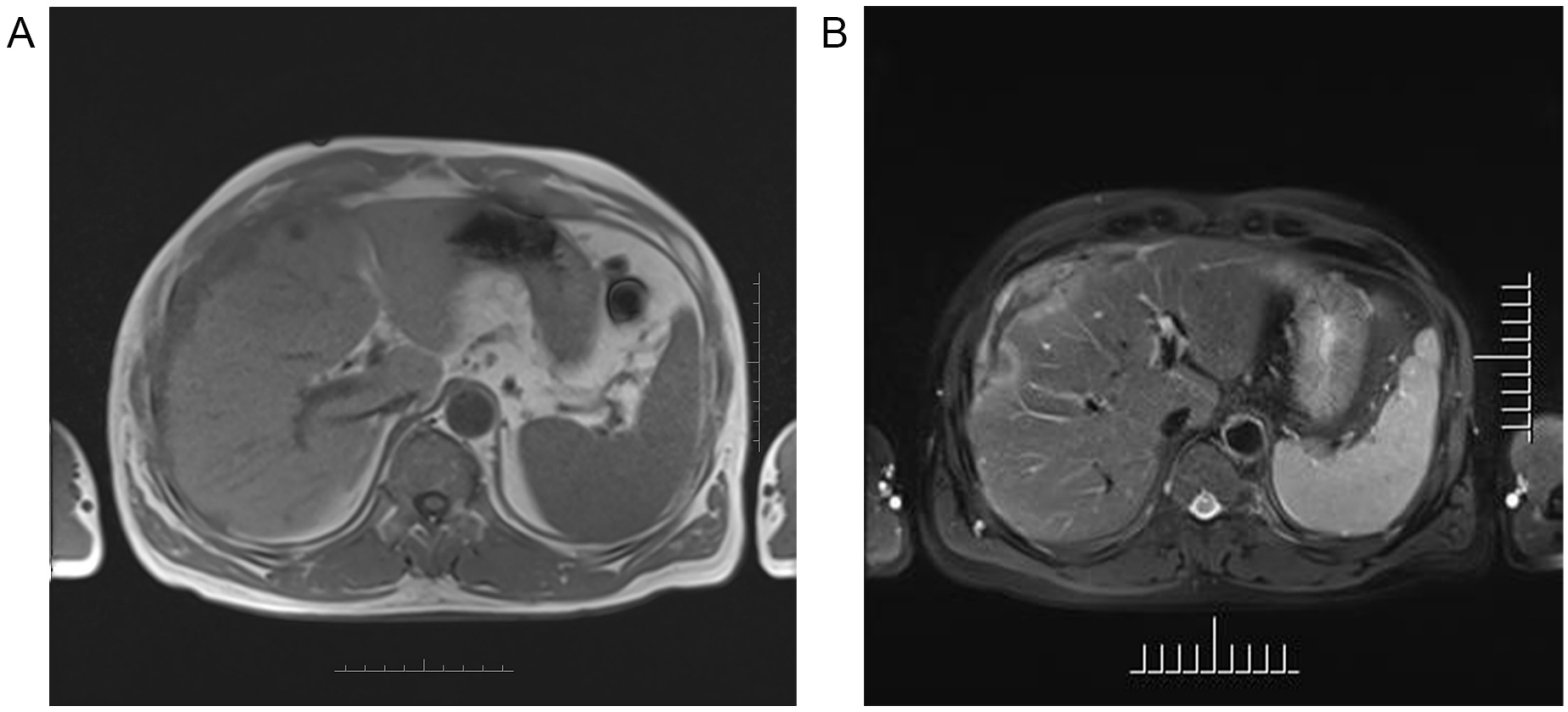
Axial MR images of the upper abdomen showing the lesion in the right subdiaphragm and right anterior and left medial lobe of the liver demonstrated intermediate-to-low intensity on T1-weighted images (A), and central intermediate-to-low intensity and peripherally intermediate-to-high signal on T2-weighted image (B).
